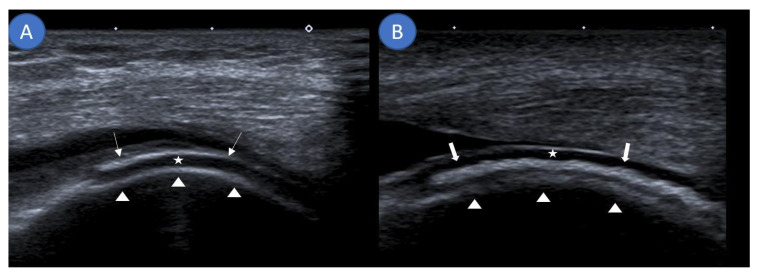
Differentiating the double contour sign from an intracartilaginous hyperechogenicity. (A) Sagittal ultrasound demonstrating monosodium urate deposits (arrows) as a hyperechoic band running parallel to the echo-free hyaline cartilage (star) at the suprapatellar knee joint. Together with the hyperechoic margin created by the femur (arrow heads), this forms the double contour sign. (B) Sagittal ultrasound along the lateral femoral condyle showing an intracartilaginous hyperechogenicity (thick arrows) visualizing calcium pyrophosphate deposits consistent with the intracartilaginous hyperechogenicity sign.
